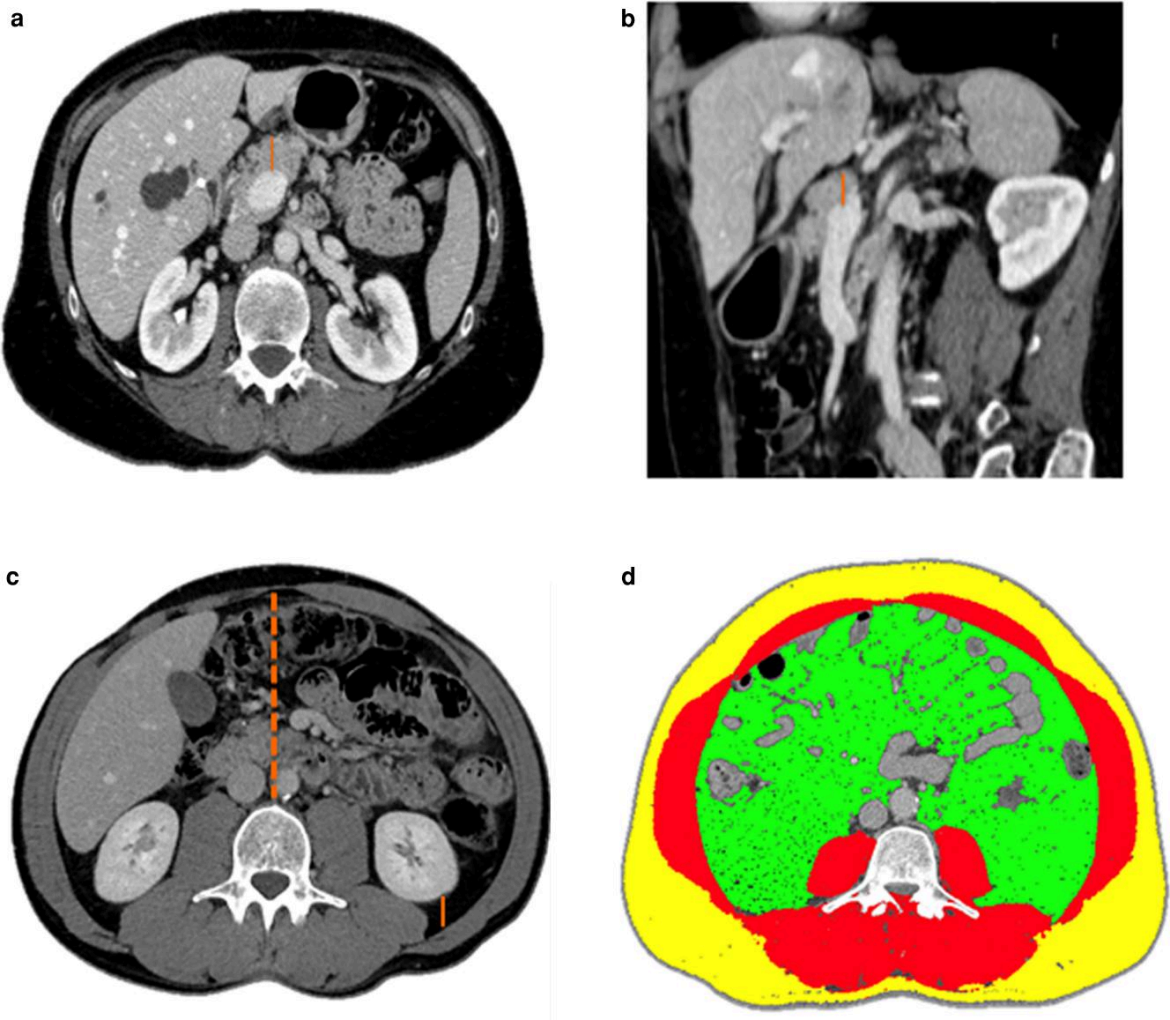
Assessment of quantitative CT parameters a On axial CT images, the anteroposterior (minor) pancreatic parenchymal thickness (orange line) was measured at the level of the splenomesenteric venous confluence. b The pancreatic parenchymal craniocaudal (major) thickness was identified on sagittal images along the maximum length of the gland. c On axial CT images, perirenal fat thickness (continuous orange line) was measured as the anteroposterior distance between the left posterior renal capsule and the adjacent junction of the posterior abdominal wall and corresponding paraspinal musculature. Intra-abdominal fat thickness (orange dashed line) was defined as the anteroposterior distance between the linea alba and the anterior surface of the third lumbar vertebra body. d Image postprocessing for the assessment of body composition parameters on axial CT at the level of the third lumbar vertebra. The subcutaneous fat area is highlighted in yellow, total abdominal muscle area in red, and visceral fat area in green.
